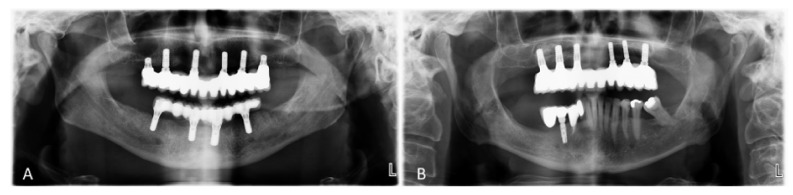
Panoramic X-ray after treatment. Short (A) and control implants (B) were loaded with fixed full-arch fixed dental prostheses (FDPs). Bilaterally, the most posterior implants were 4 mm long (A) or 10 mm long (B) that were installed after sinus floor augmentation.
